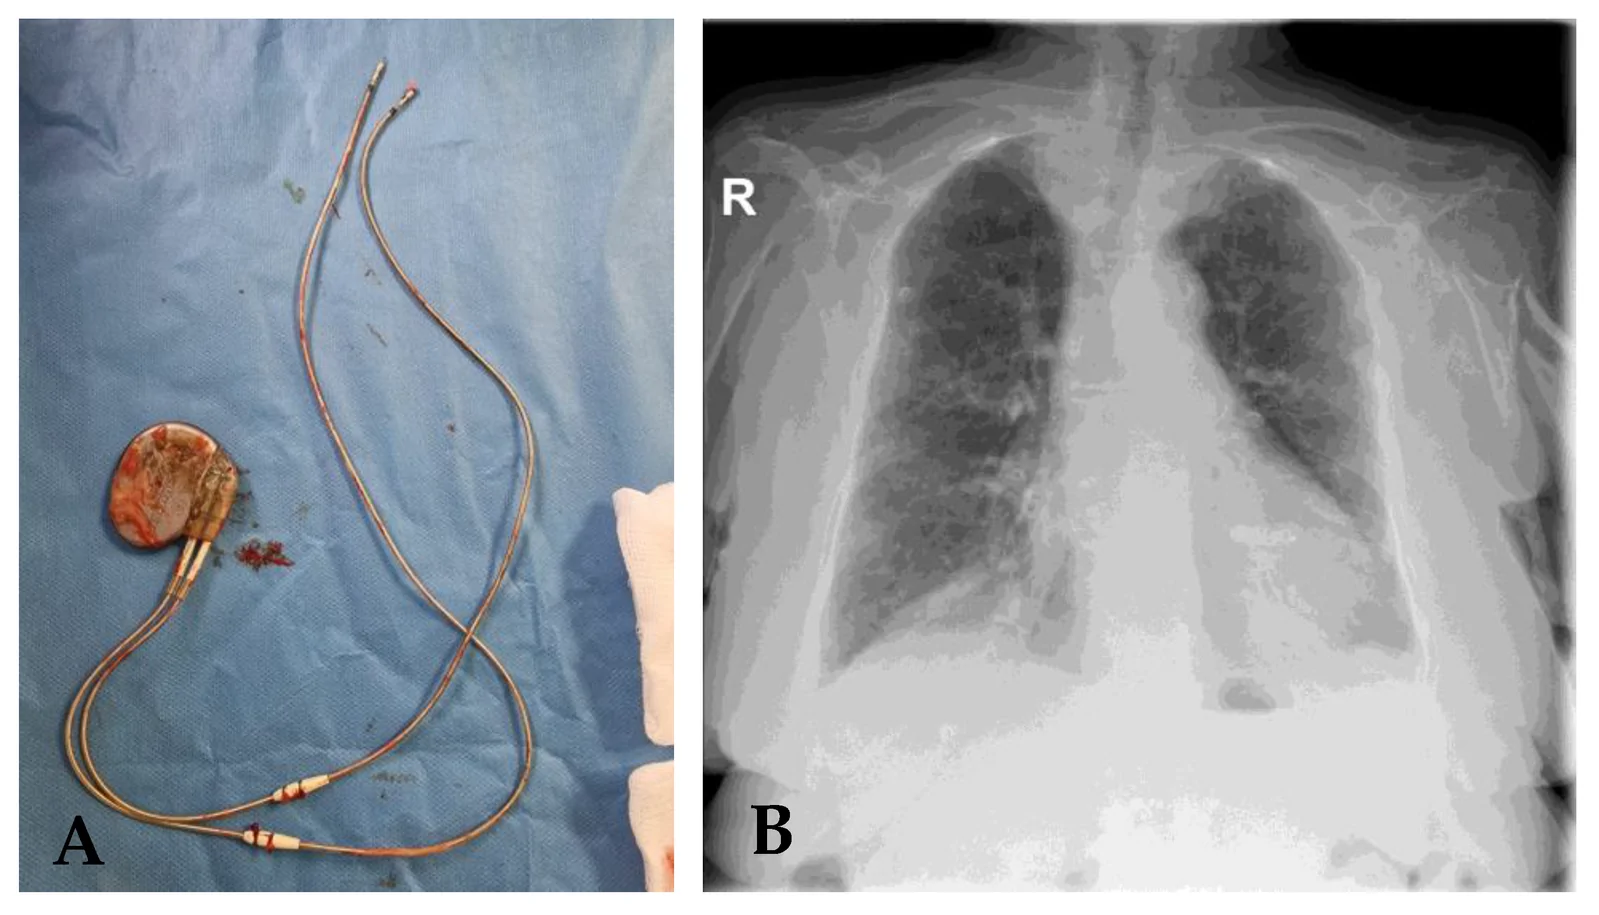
(A) The explanted pacemaker and four-year-old leads (by simple manual traction). (B) Posteroanterior (PA) chest X-ray showing the placement of a Micra™ VR, Medtronic, Minneapolis, MN, USA, leadless transcatheter pacemaker.
